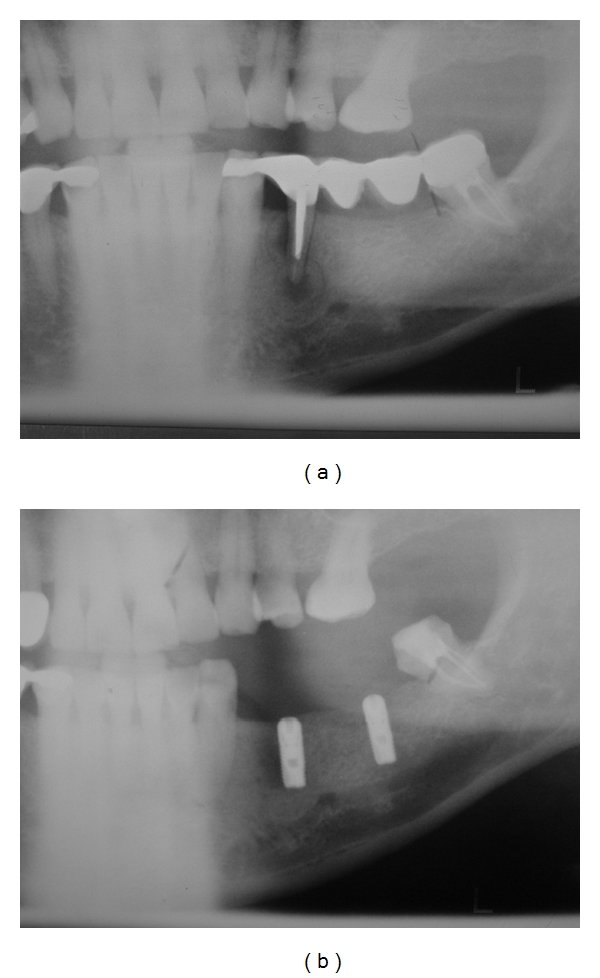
(a) Panoramic radiograph revealing periapical radiolucency in endodontically treated left mandibular premolar, when patient was completely healthy (March 2006). (b) Panoramic radiograph showing dental implants before final restoration. Implants were completely osseointegrated, and no mandibular lesions were observed (September 2006).
